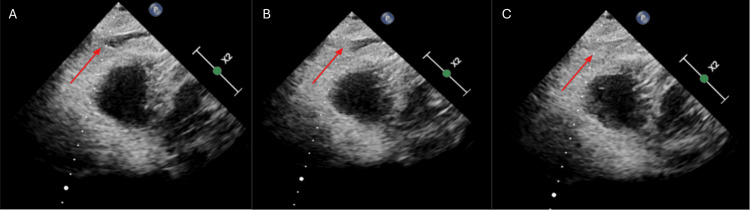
Transthoracic contrast echocardiography following microbubble injection through the catheter. (A-C) Transthoracic contrast echocardiography following microbubble injection through the catheter at three different time points.
